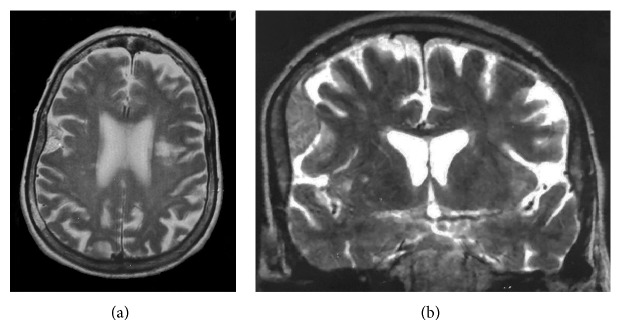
MRI images of the patient. Axial (a) and coronal (b) T2-weighted images show a hyperintense extra-axial right parietal convexity space occupying lesion, compatible with a meningioma. The skull adjacent to the lesion is thicker than contralateral bone. The separation plan is hypointense.
